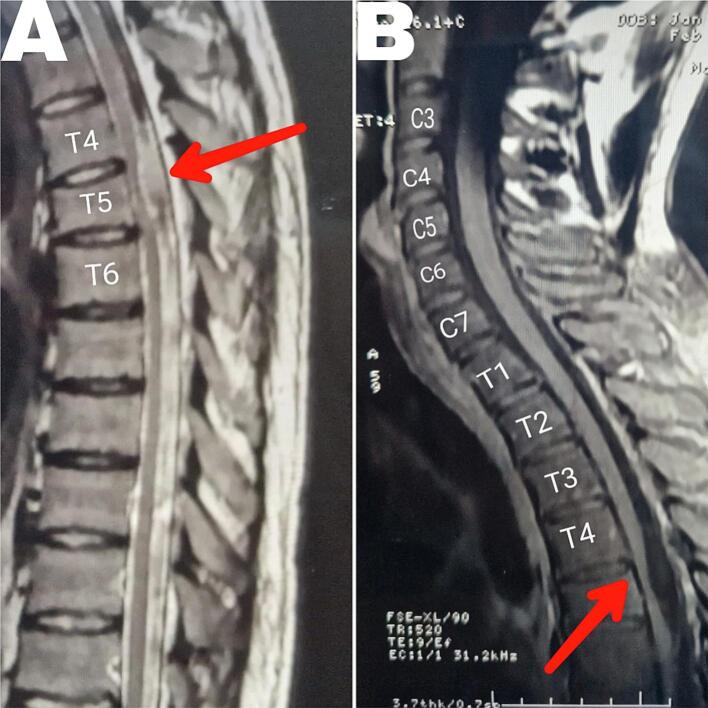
A pre-operative MRI. (A) T2 and (B) T1 show the C-shaped displacement of the thinned thoracic spinal cord, and the absence of cerebrospinal fluid (CSF) flow ventral to the herniated cord (red arrows). A hyperintense signal on the T2 in the level of the fifth Thoracic vertebra, indicates a myelopathy. (For interpretation of the references to colour in this figure legend, the reader is referred to the web version of this article.)
